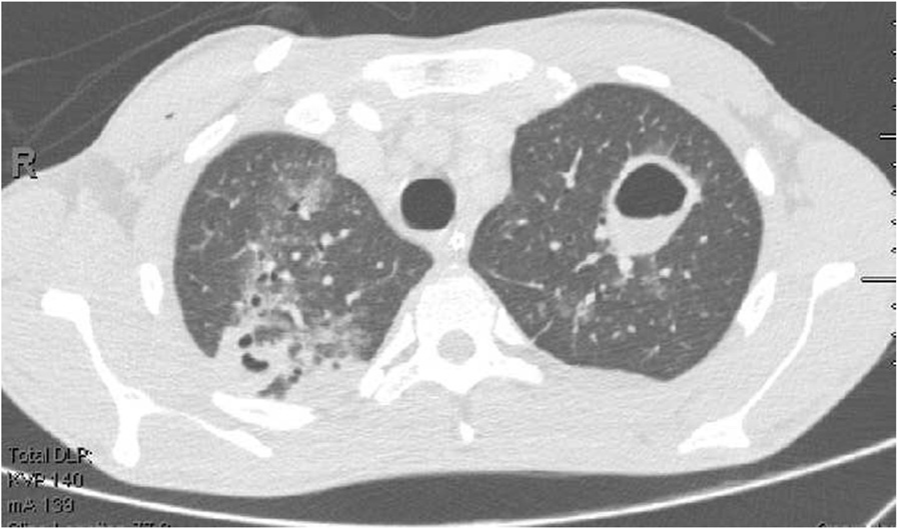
Upper third of the chest in computed tomography scan performed on the 11th day of hospitalization showed foci of pulmonary consolidation and cavities with thickened walls and fluid levels
